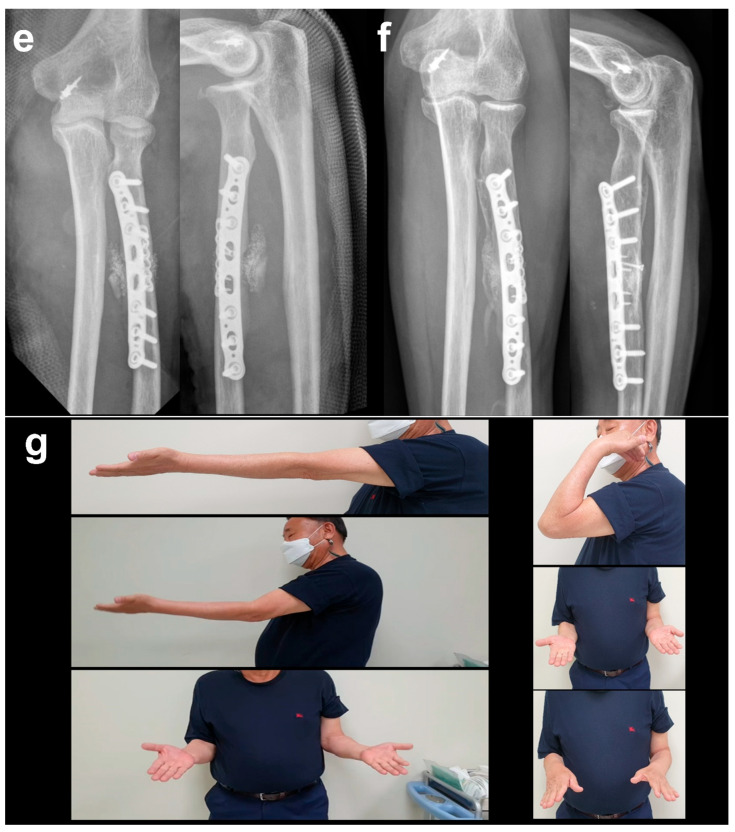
Surgical implementation of hydroxyapatite-carrier rhBMP-2. (a) A 69-year-old male patient diagnosed with Arbeitsgemeinschaft für Osteosynthesefragen/Orthopaedic Trauma Association (AO/OTA) 2R2-B3 associated with multiple wedge fragments. (b) After open reduction and plate fixation, a cortical defect on the medial side was identified (black arrow). (c) A separate medial wedge fragment (white arrow) created a cortical defect and was at risk of devitalization if anatomical reduction had been attempted. (d) rhBMP-2 was applied to the medial cortical defect and along the fracture line between the stripped wedge fragment and the distal diaphysis (white arrow). (e) Postoperative radiograph showing fixation with a 3.5 mm locking plate and a 2.0 mm reduction plate. A hydroxyapatite (HA)-based carrier system containing 0.5 mg of rhBMP-2 is visible on the medial, lateral, and dorsal aspects of the ulnar fracture site. (f) Fracture healing was achieved without evidence of heterotopic ossification or radioulnar synostosis. The grafted material was resorbed during the fracture healing process. (g) The patient achieved a favorable functional outcome without any significant limitation in range of motion.
